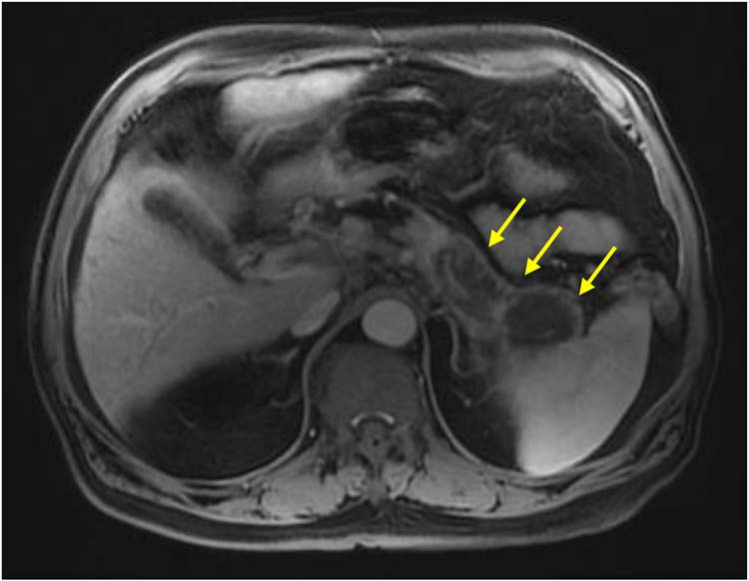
MRI abdomen (MRCP) with IV contrast: axial image showing diffuse enlarged hypointense signal of pancreatic body and tail on T1 weighted imaging encircled by a thin rim of enhancement representing normal pancreatic tissue (arrows).
